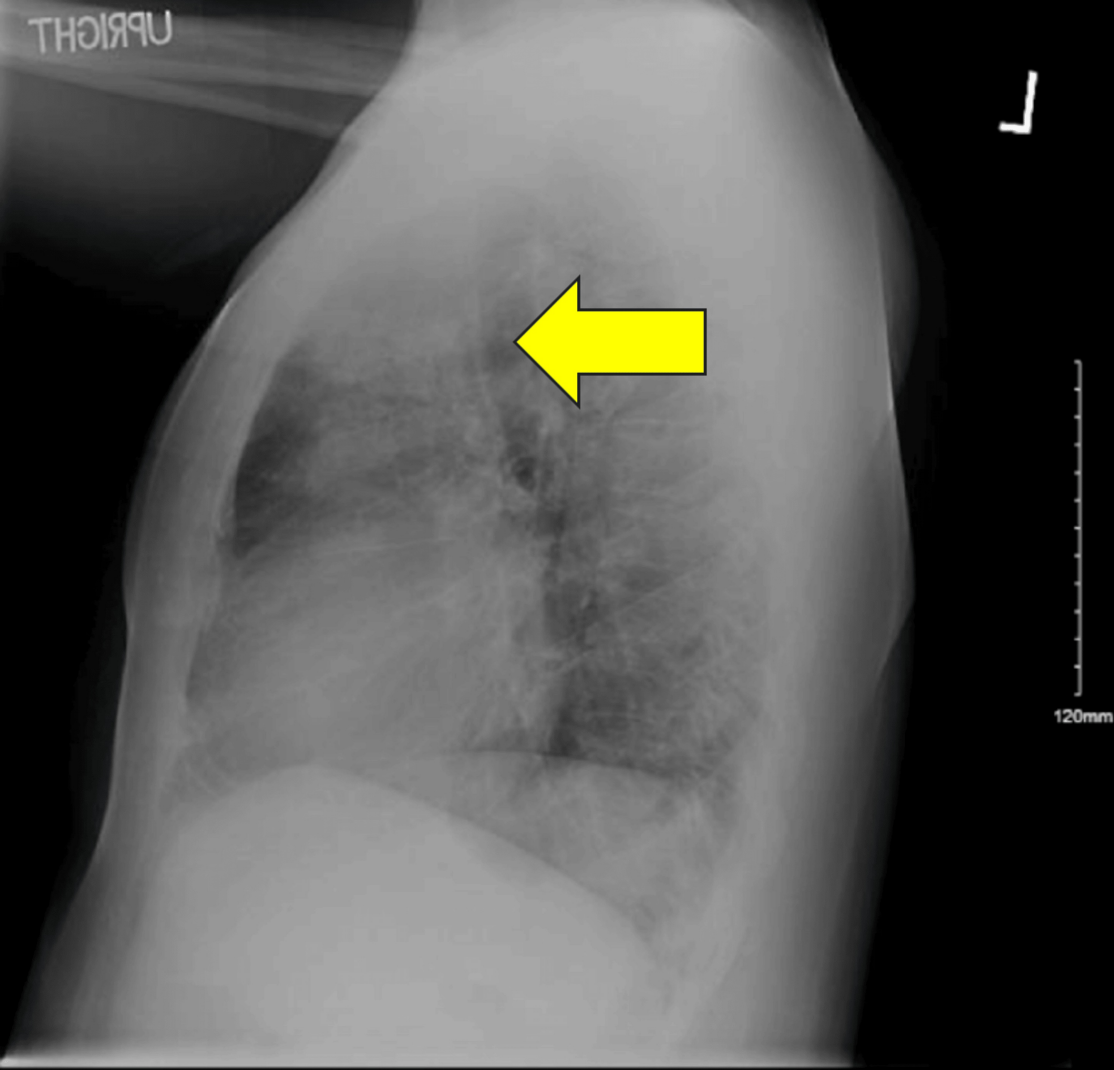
Lateral view of chest X-ray demonstrating patchy airspace disease in the left upper lobe.
